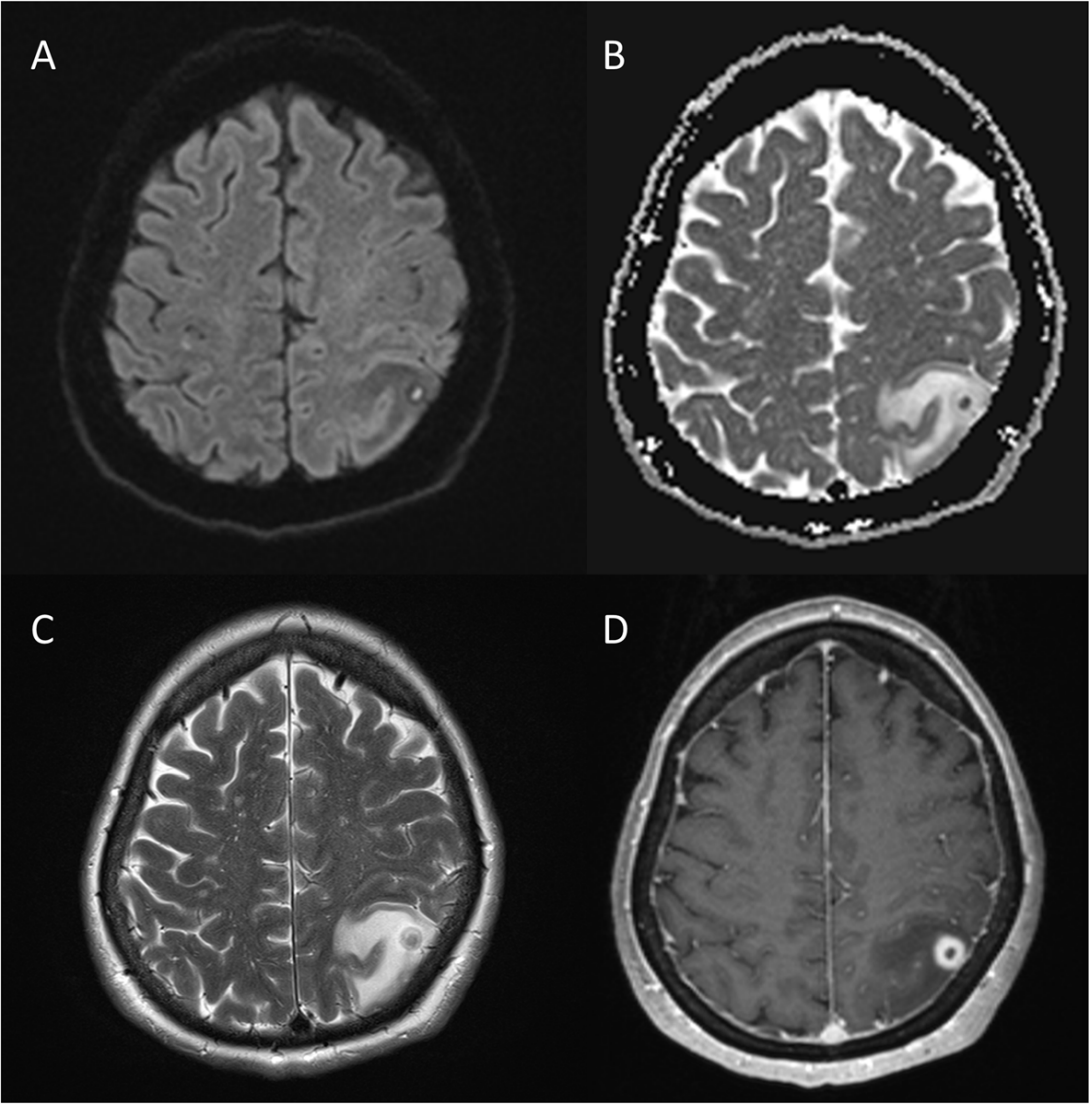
Pre-operative axial MRI findings. Diffusion-weighted images (a), with corresponding ADC-map (b), showed a diffusion restricted left parietal lesion. On T2-weighted images (c) the lesion appeared T2-hyperintense, with both a T2-hypointense central region and thin rim. The lesion showed intense ring-enhancement in the T1-weighted contrast-enhanced images (d) and was surrounded by marked perifocal edema (c)
